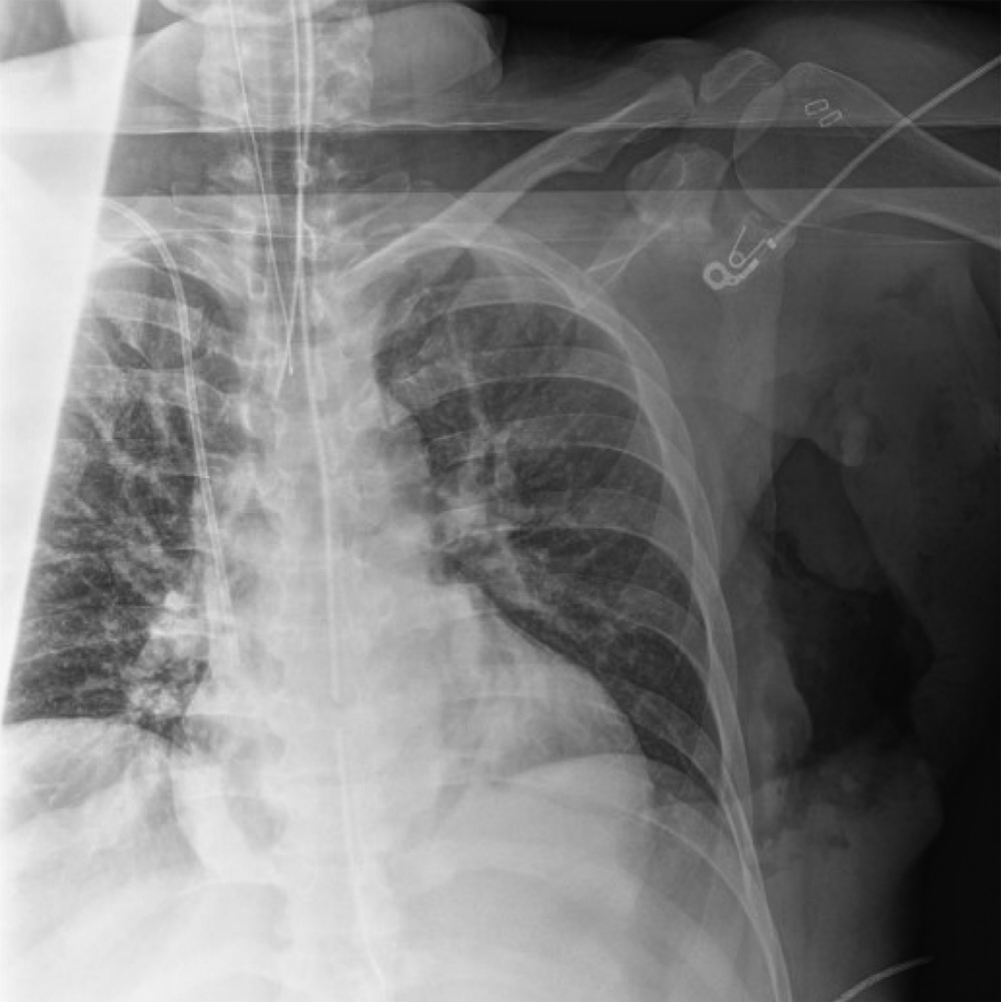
Intraoperative X-ray of chest and axilla. Intraoperative radiograph of the patient's chest and axilla did not visualize a lymph node containing a clip in-vivo.
